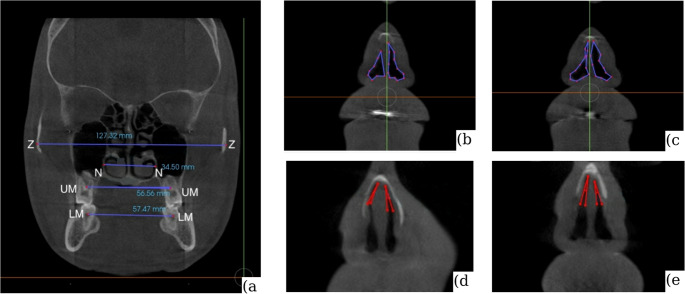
Landmarks and measurements on coronal CBCT scans: (a) reference landmarks: Z, most lateral point of zygomatic arch; N, most lateral point of nasal cavity; UM, furcation of upper first molar; LM, furcation of lower first molar. (b) INV cross-sectional area, preoperative. (c) INV area, postoperative. (d) INV angle, preoperative. (e) INV angle, postoperative
